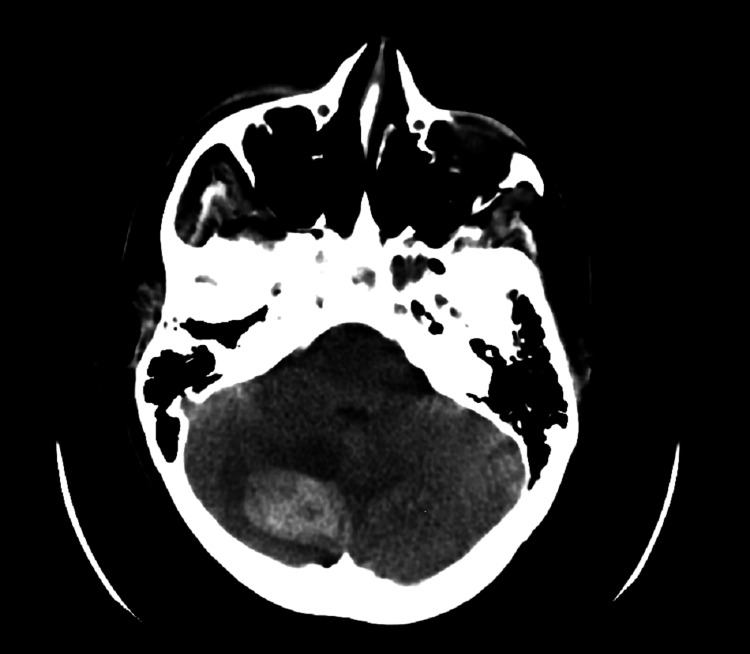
Brain CT showing a remote cerebellar hemorrhage.
